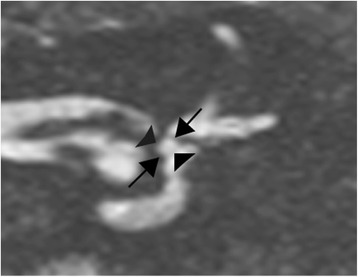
3T MRI. Coronal T2-weighted gradient-echo FIESTA-C image, through the anterior and external ampullas. Example of the measurements of the saccular height (arrows) and width (arrowheads)the saccule was measured from the middle of the lateral wall to the inner part of the medial wall of the adjacent vestibule, as shown in Fig. 2.
